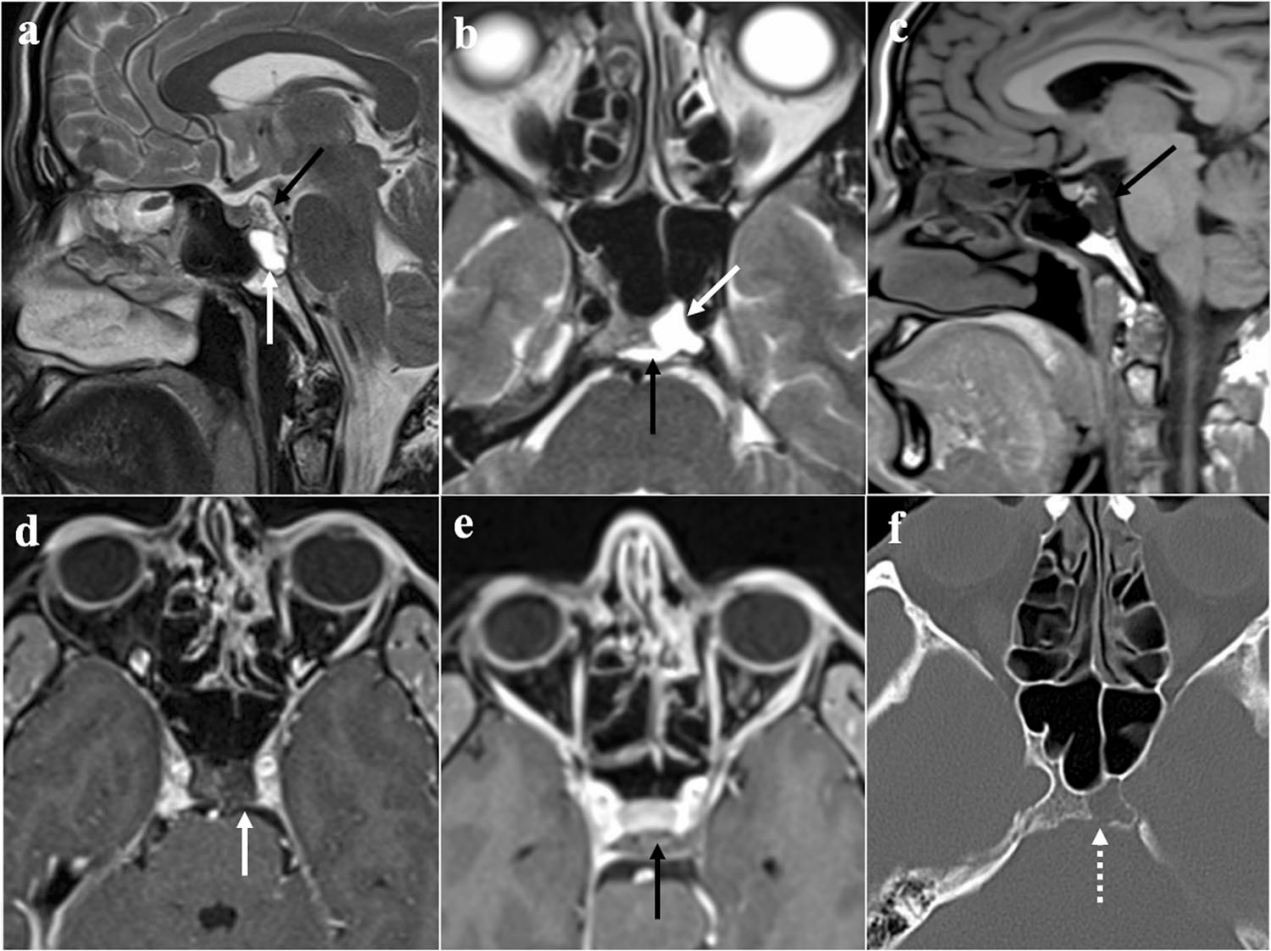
Ecchordosis physaliphora in a 24-year-old male patient with headache. MRI shows a midline, intradural, cystic lesion located in the retroclival prepontine region (black arrows) with intraosseous extension into the dorsal aspect of the clivus (white arrows). It shows T2 high (a, sagittal; b, axial), T1 low SI (c, sagittal), and lack of enhancement after gadolinium contrast media intravenous injection (d, e, axial). CT (f) reveals a bony defect in the dorsal clivus representing the stalk (white dotted arrow) connecting the retroclival and intraosseous components of the lesion. Note the well-marginated and scalloped bone margins of the lesion in the dorsal clivus
